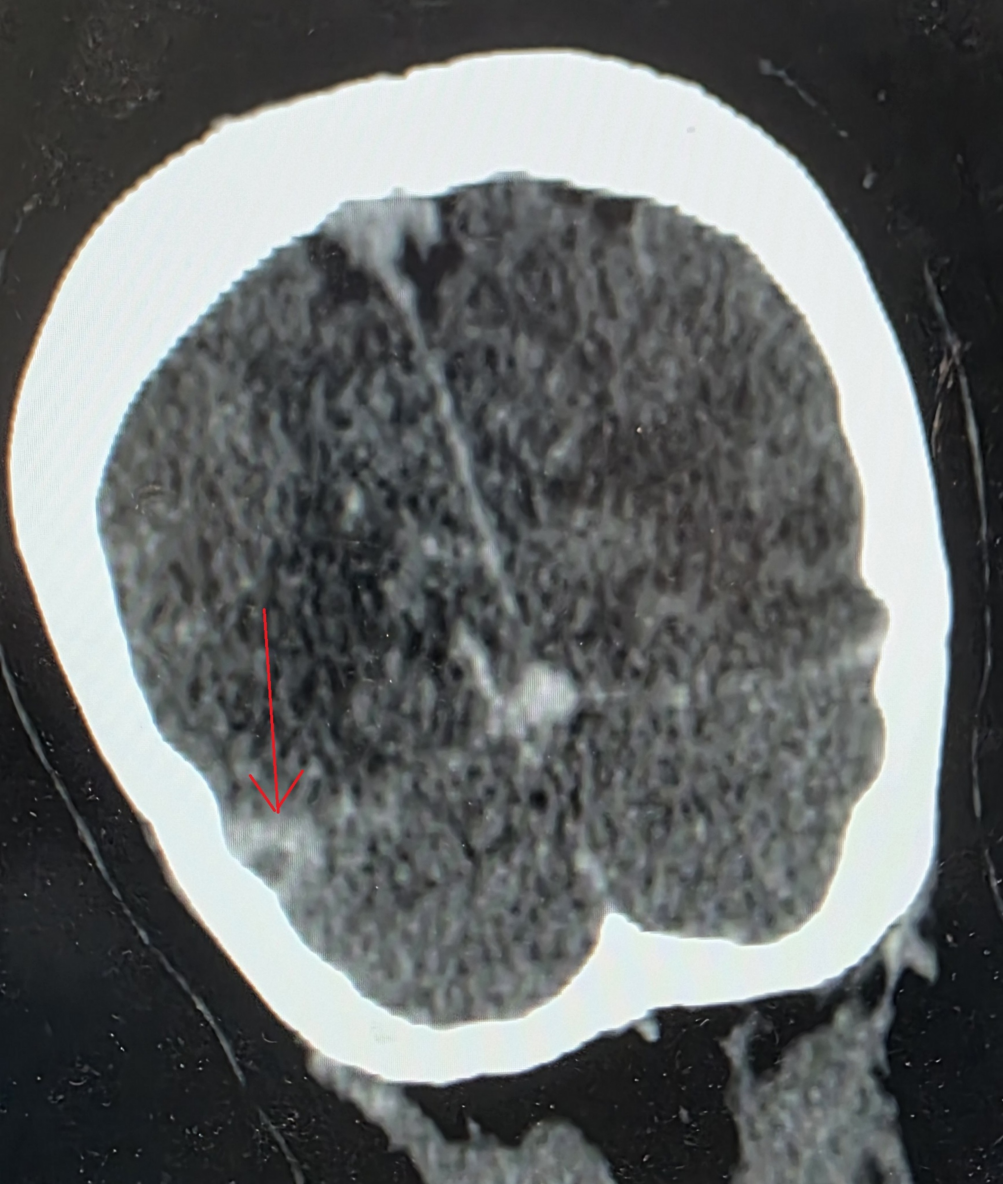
Contrast-enhanced axial brain CT showing the empty delta sign (arrow) CT: computed tomography
